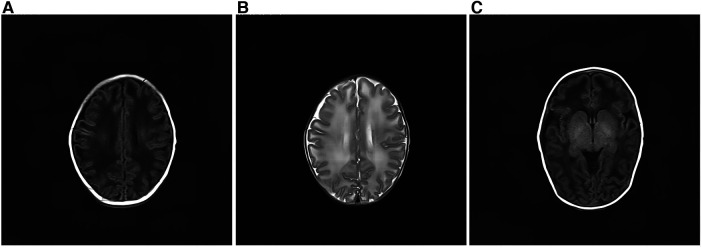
Cranial magnetic resonance imaging of the patient. (A) There was a wide range of long T1 signals in the bilateral frontotemporal parietal lobes. The lesions on both sides are roughly symmetrical. (B) The bilateral frontotemporal parietal lobes had a wide range of long T2 signals. The lesions on both sides are roughly symmetrical. (C) Short T1 signals can be seen in the bilateral pallidum.
